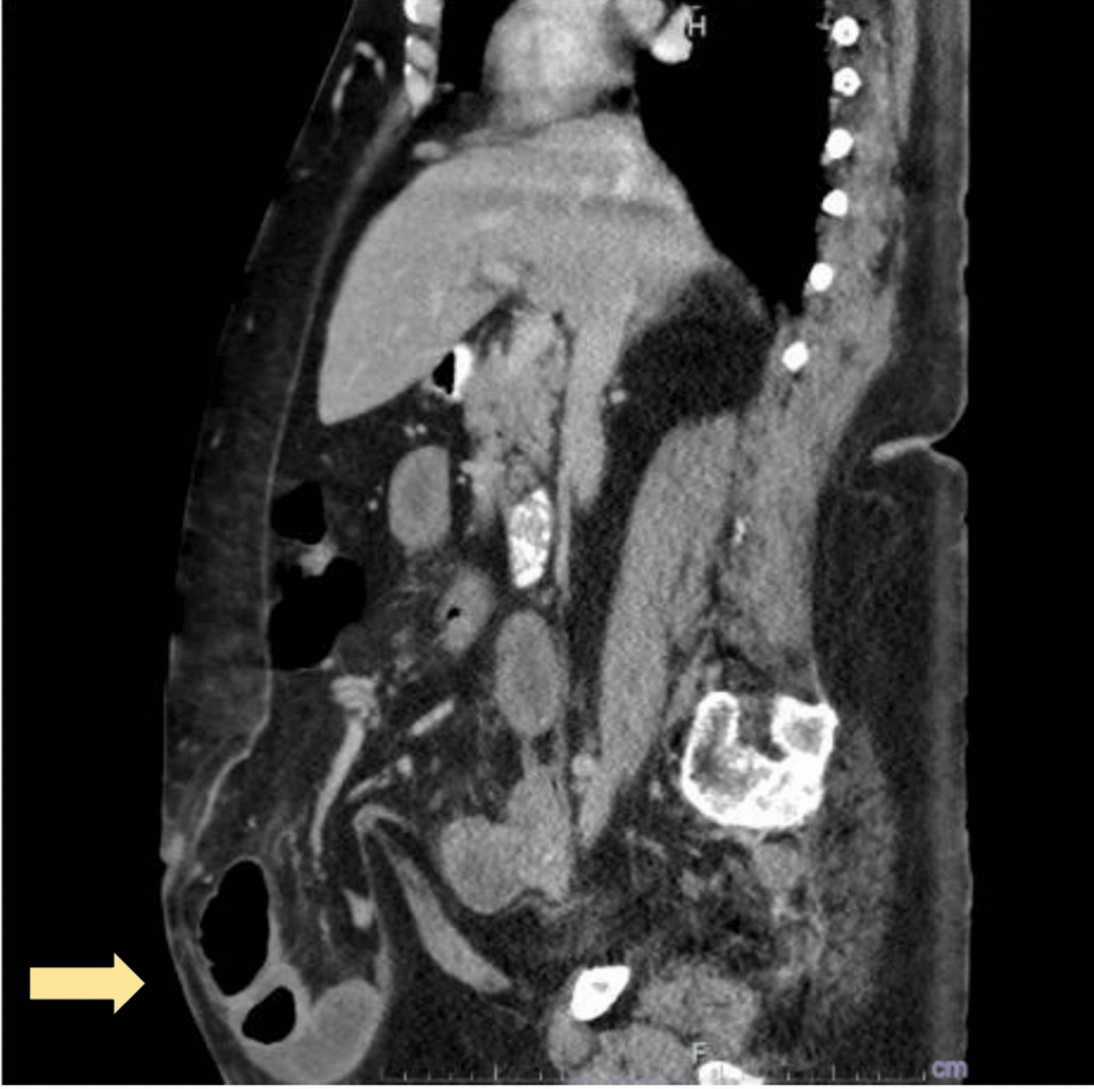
CT abdomen (coronal view) The image shows incisional hernia with thickened hernia sac with small bowel content (yellow arrow) CT: computed tomography
